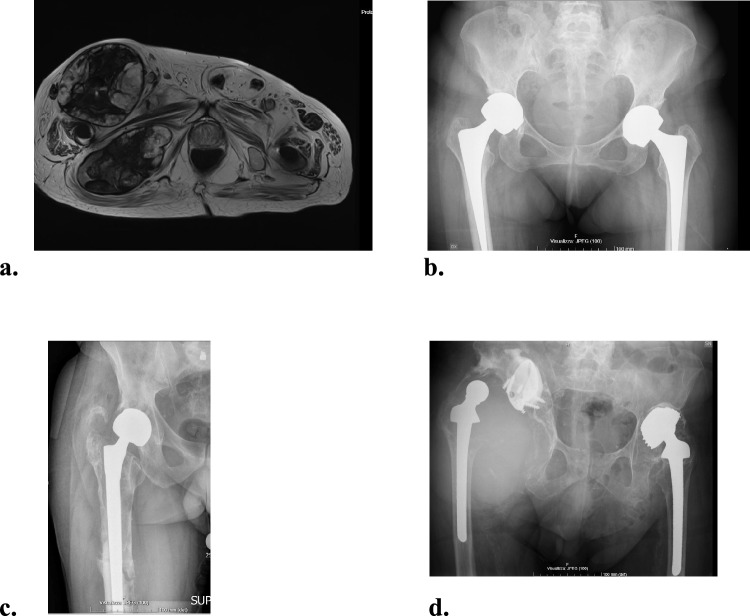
a–d MRI T2-weighted axial view reveals a large intra- and extrapelvic pseudotumor, classified as Anderson Grade C2, Matthies Class III, and Hauptfleisch Type III. These classification systems do not address implant status. Images b–d highlight potential scenarios linked to the pseudotumor, each posing unique technical challenges and reconstruction requirements
